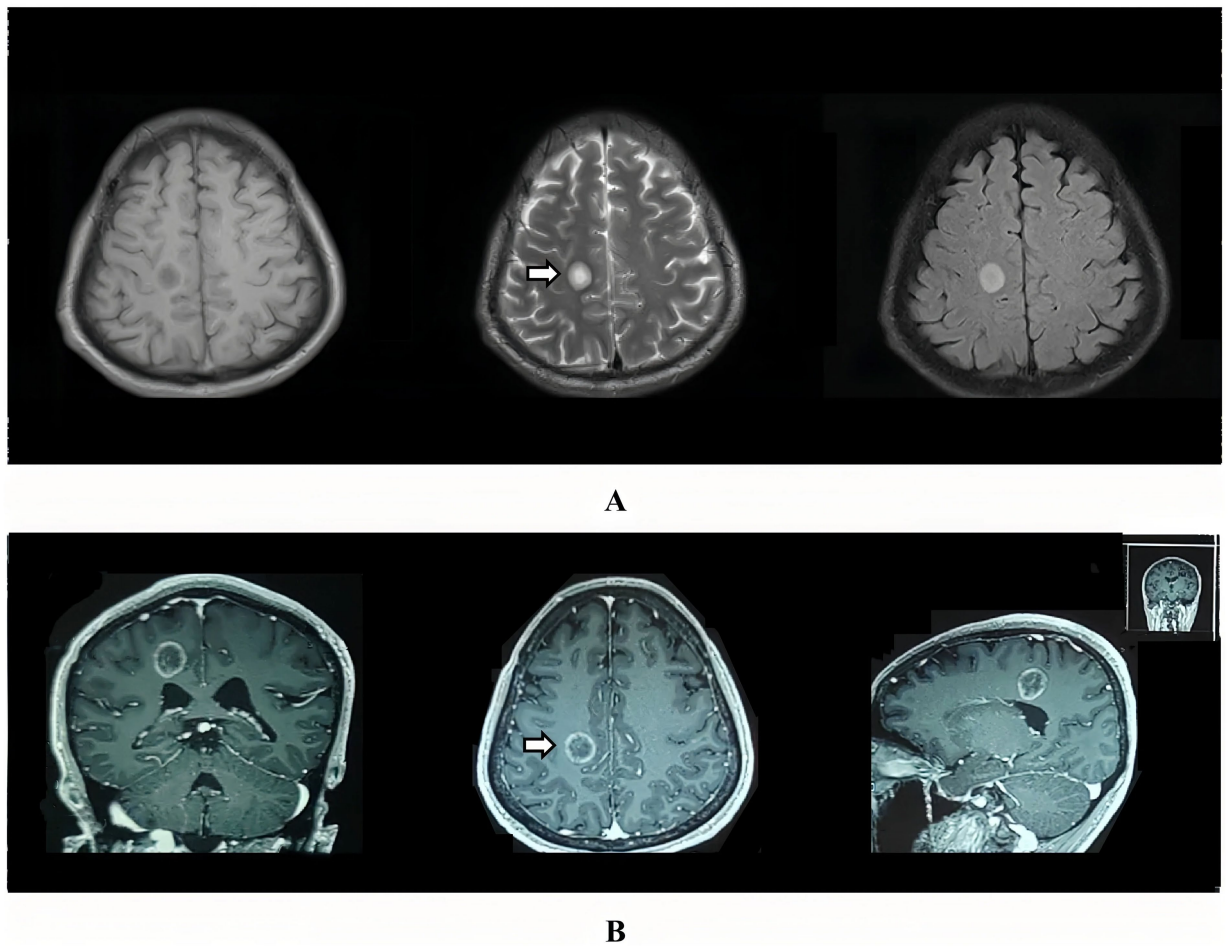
Serial brain MRI findings. (A) Axial T1-weighted, T2-weighted, and FLAIR sequences demonstrated demyelination-like signal abnormalities in the right centrum semiovale and left parietal lobe. White arrows indicate the core demyelinating lesions. (B) Gadolinium-enhanced coronal, axial, and sagittal T1-weighted images revealed characteristic “onion-bulb” concentric ring-like enhancing lesions, featuring alternating T2/FLAIR hyperintense-isointense rings (consistent with the “fried egg” sign in Balo’s concentric sclerosis). White arrows indicate the pathognomonic concentric ring lesions.
